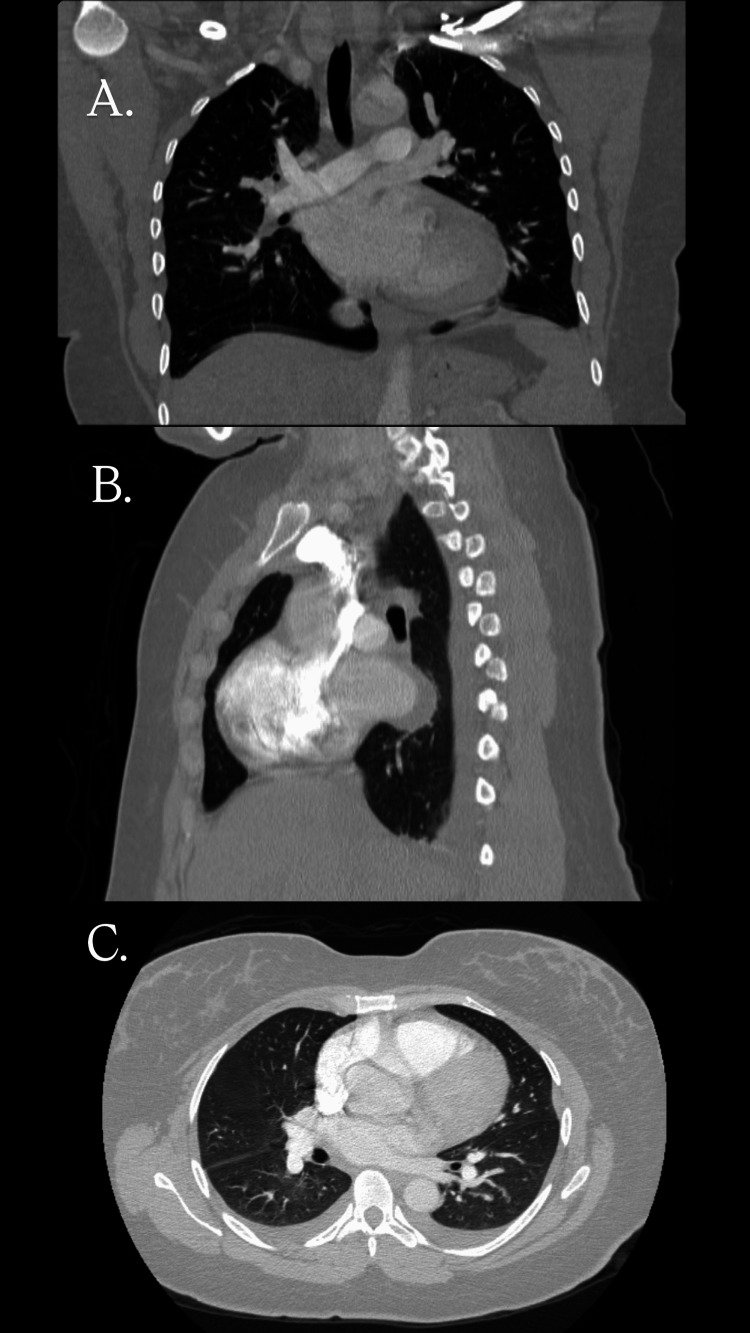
Computed tomography (CT) of the chest with intravenous contrast. (A) Coronal view demonstrating an enlarged cardiac silhouette with mild bilateral pleural effusions. (B) Sagittal view highlighting the enlarged heart relative to the thoracic cavity. (C) Axial lung window image showing small bilateral pleural effusions without focal consolidation. No evidence of pulmonary embolism was identified.
